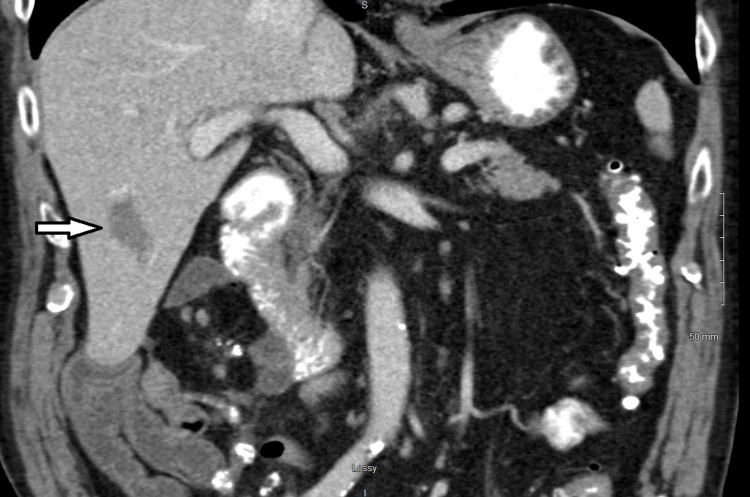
CT after RFA to biopsy-confirmed liver OM (white arrow) in 2012 CT, computed tomography; OM, oligometastases; RFA, radiofrequency ablation
